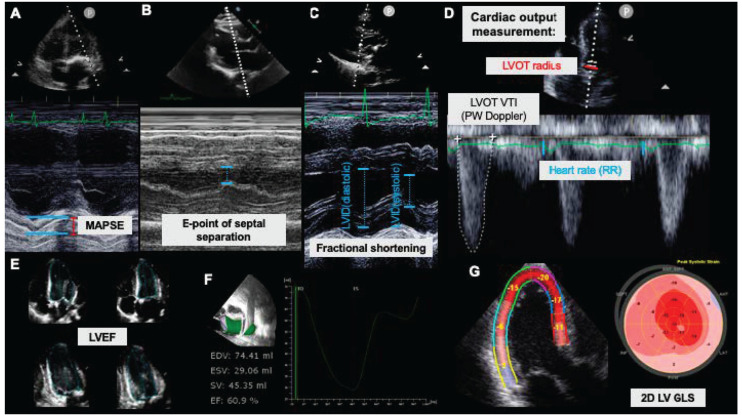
Echocardiographic evaluation of left ventricular systolic function. (A) Measurement of mitral annular plane systolic excursion (MAPSE, mm) using M-mode in transthoracic apical four-chamber view. (B) Quantification of the E-point of septal separation during early diastolic inflow in the transthoracic parasternal long-axis plane. (C) Fractional shortening is measured in the long-axis view and reflects the systolic circumferential function of the left ventricle. (D) By measuring the velocity time integral (VTI) with the PW Doppler in the left ventricular outflow tract (LVOT) while including the LVOT radius and heart rate (RR interval) into the calculations, the cardiac output can be quantified noninvasively. (E) The Simpson method derives LV volumes and left ventricular ejection fraction (LVEF) from four- and two-chamber views. This requires tracing of the endocardial border in the end-diastolic and end-systolic frames. (F) Three-dimensional echocardiographic acquisition of the entire left ventricle provides accurate volumetry and LVEF measurement. The technique is usually combined with semi-automatic speckle-tracking to allow for quick and robust analyses. (G) Speckle-tracking is further applied to 2D long-axis views of the left ventricle to measure longitudinal strain. The 2D LV global longitudinal strain of the LV (GLS) is a parameter with a better prognostic power compared to LVEF. EDV: end-diastolic volume; ESV: end-systolic volume; LVID: left ventricular inner diameter; SV: stroke volume.
